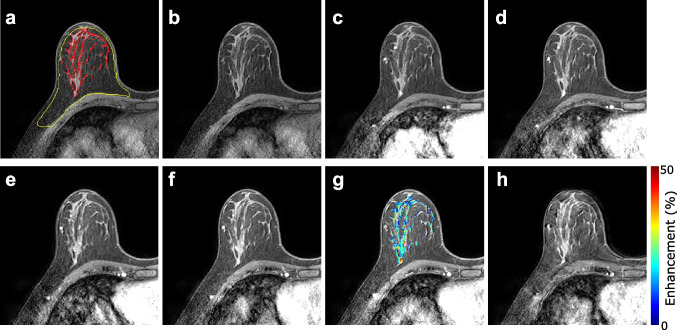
Segmentations generated from T2-weighted data were applied to DCE-MRI data (a) across all time points. The sequence of DCE-MRI images taken before (b) and after (c–h) bolus injection of the contrast agent is shown for an example patient. An enhancement map is shown on the 5th post-contrast image (g), which was the image volume with the highest median pixel value over the segmented parenchyma. Post-contrast images were acquired every 59.1 s
